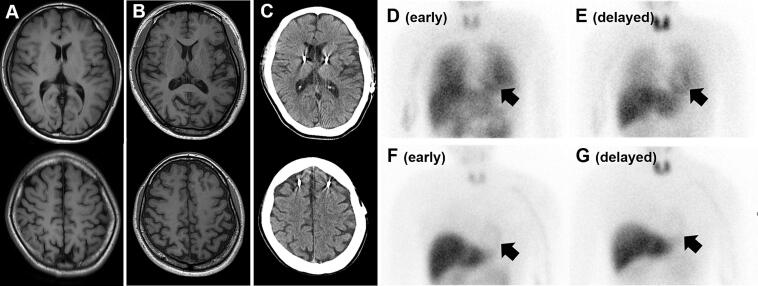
(A–C) Serial brain imaging showing no significant cerebral atrophy: T1-weighted MRI at 25 (A) and 31 (B) years of age, and CT at age 36 (C). (D–G) ^123^I-MIBG myocardial scintigraphy. Initial scans at age 29 (D, E) show normal cardiac uptake (heart-to-mediastinum [H/M] ratio: early, 3.22; delayed, 3.35). Follow-up scans at age 36 (F, G) reveal markedly decreased uptake (H/M ratio: early, 1.92; delayed, 1.79). MRI, magnetic resonance imaging; CT, computed tomography; H/M heart-to-mediastinum; MIBG, metaiodobenzylguanidine.
